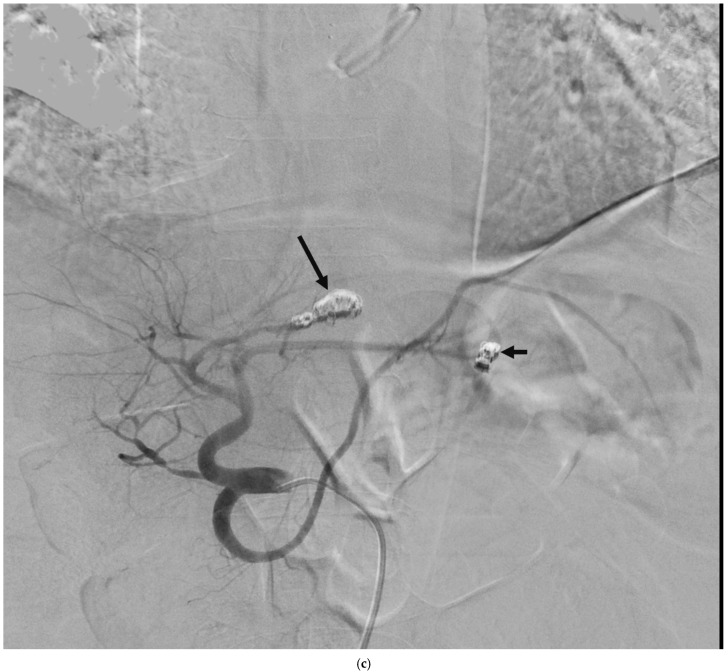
Two hepatic artery infectious aneurysms in a *Streptococcus sanguinis* infectious endocarditis. A 53 year-old man, presented a *Streptococcus sanguinis* infectious endocarditis on Bentall tube, and concomitant splenic infarct and ruptured intracranial aneurysm. Abdominal CT-angiography (CTA) showed two distal and fusiform infectious aneurysms of the left hepatic artery (left liver, segment II) of respectively 12 ((a), long arrow) and 4 mm ((a), short arrow) in diameter. These aneurysms were evidenced on selective angiography (respectively, (b), long arrow and short arrow). Both were excluded by coil embolization (respectively, (c), long arrow and short arrow).
